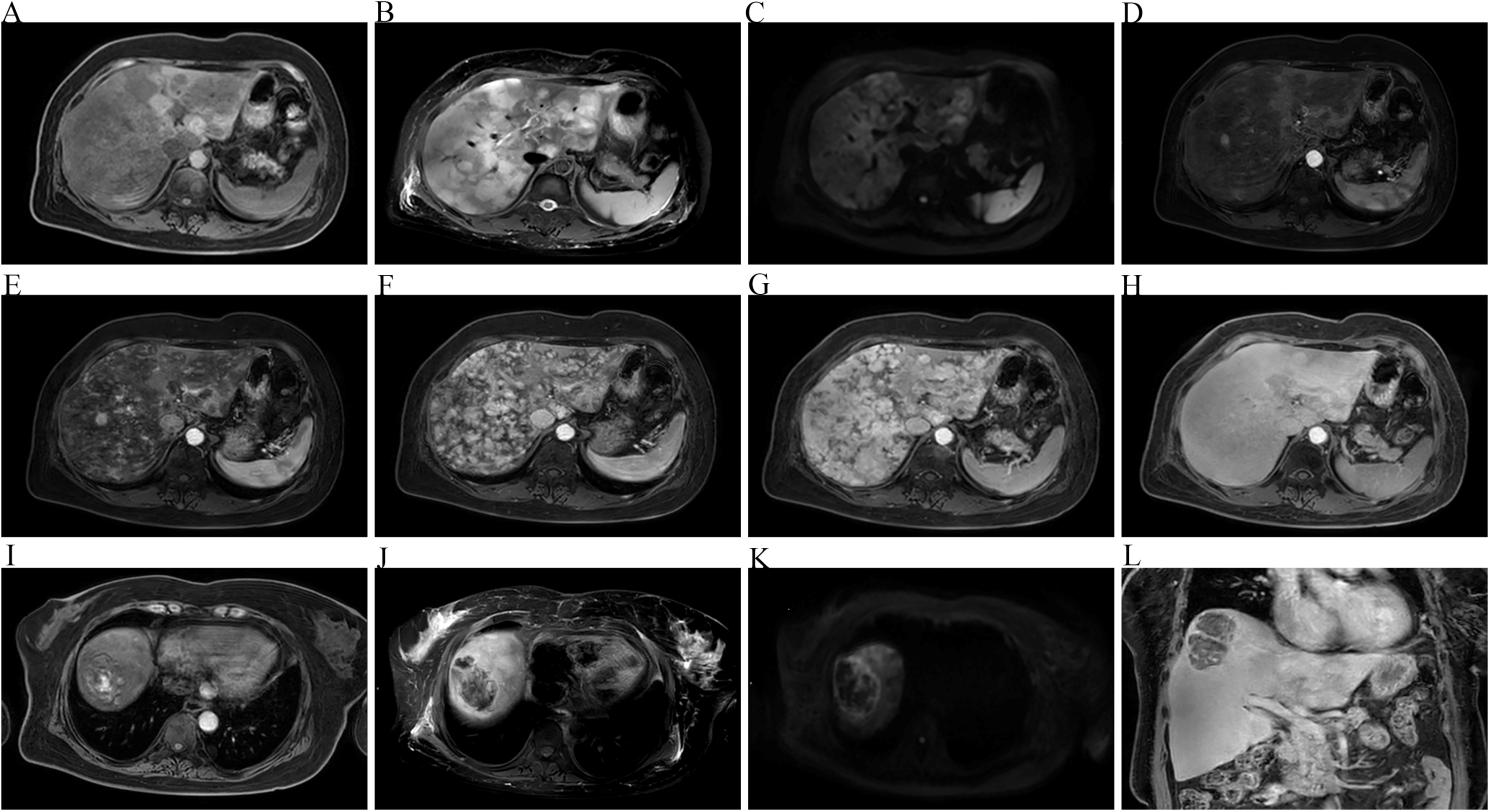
(A–C) Multiple lesions showing hypointensity on T1-weighted images (T1WI) (A) and hyperintensity on T2-weighted images (B) and on diffusion-weighted imaging (DWI) (C). (D, E) Lesions heterogeneously enhanced in the arterial phase. (F, G) Lesions progressively significantly enhanced in the portal phase (F) and the delayed phase (G), with multiple nodular hyperintensity lesions. (H–L) No hypoenhancement was evident in the hepatobiliary phase (H, L), except for one hemorrhagic lesion (I–L).
